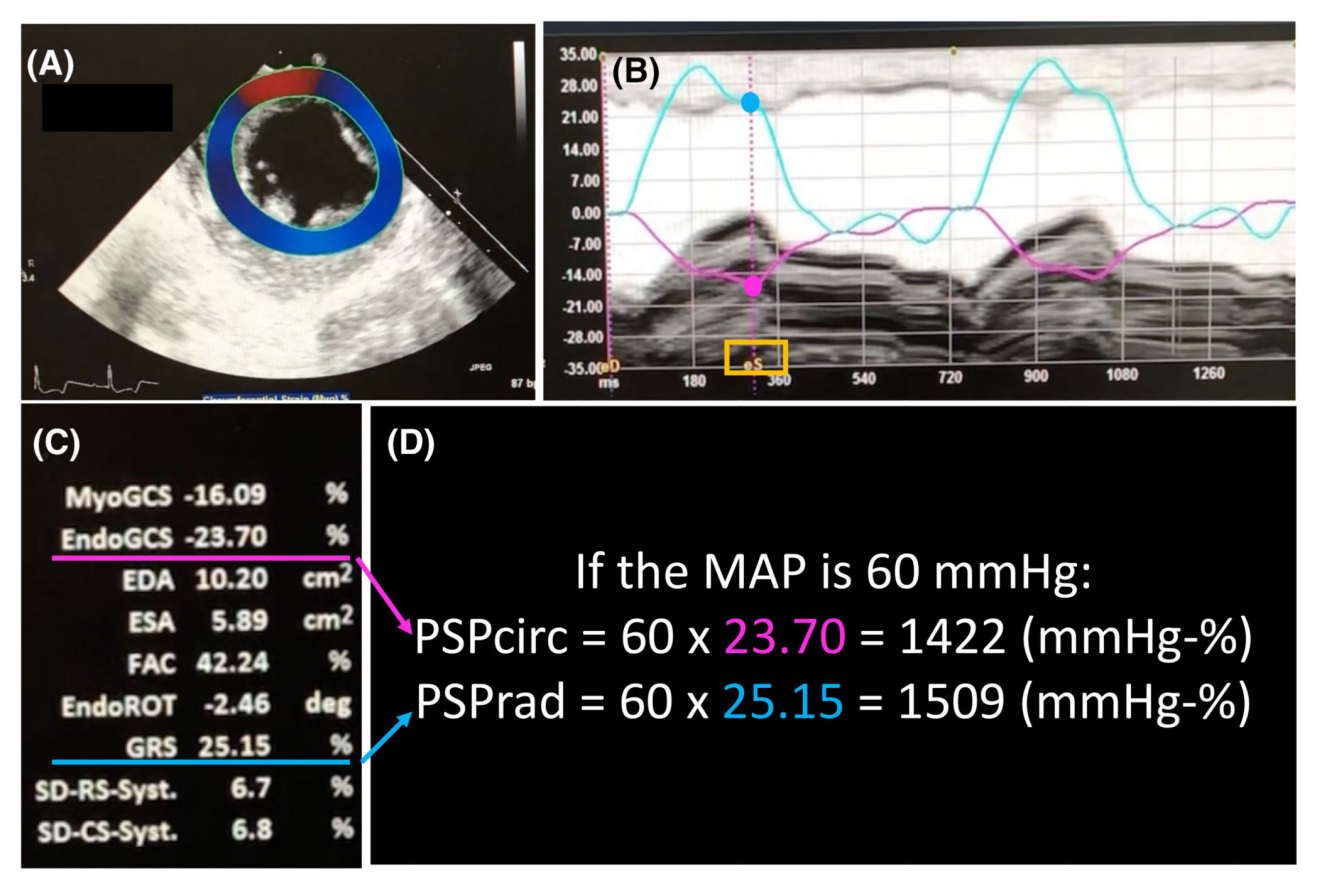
An example of image for the measurement of PSPcirc and PSPrad. (A) Describes LV short axis view at mid-papillary level showing the myocardial layer (dark blue). (B) Describes two strain curves: endo-myocardial GCS (pink) and GRS (light blue). Each value at the end-systolic phase (eS, highlighted in yellow) was used for the calculation of PSPcirc and PSPrad. (C) Describes echocardiographic parameters including myocardial and endocardial GCS, EDA, ESA, FAC, GRS and delta-rotation as the average value from the basal, mid-papillary, and apical levels. (D) Describes the formula for calculating PSPcirc and PSPrad using endocardial GCS and GRS respectively. EDA, end-diastolic area; ESA, end-systolic area; FAC, fractional area change; GCS, global circumferential strain; GRS, global radial strain; MAP, mean arterial pressure; PSPcirc, pressure strain product based on GCS; PSPrad, pressure strain product based on GRS; ROT, rotation; SD-CS, standard deviation of circumferential strain; SD-RS, standard deviation of radial strain.
